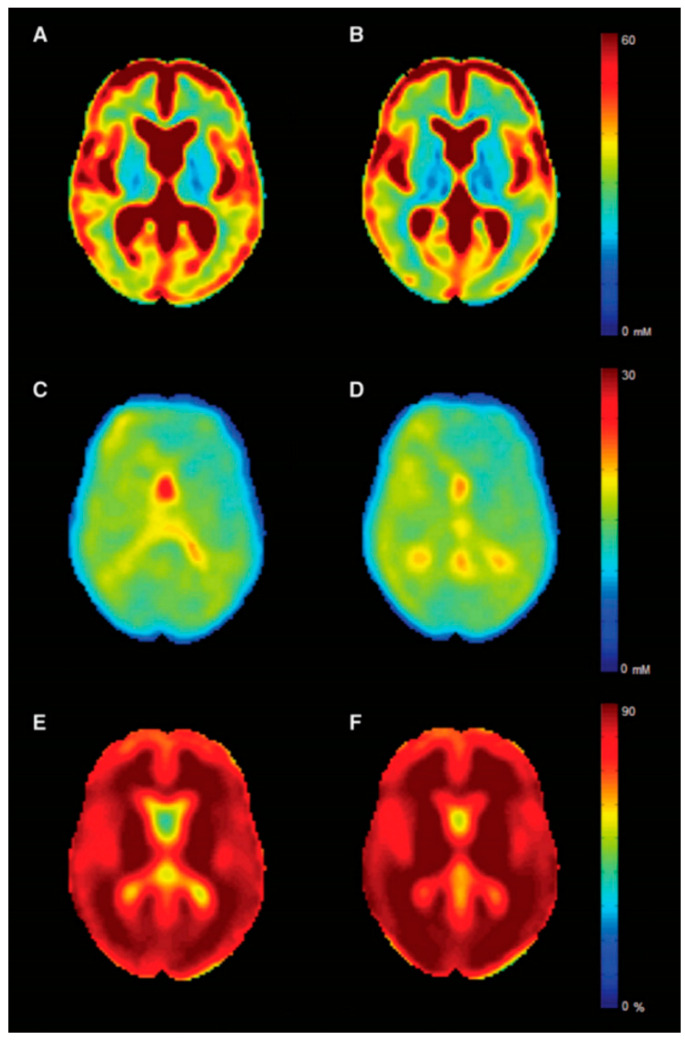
Sodium maps. Mean sodium MRI maps for multiple sclerosis patients (left column) and controls (right column). In the first row is displayed the total sodium concentration (A,B), while in the middle and last rows, the intracellular sodium concentration (C,D) and the intracellular sodium volume fraction (indirect measure of extracellular sodium concentration) (E,F) are shown. Reprinted from *Brain*, Vol. 139, Issue 3, P 795–806, 20 January 2016 [167]. Copyright: © 2021] with permission from Oxford University Press.
